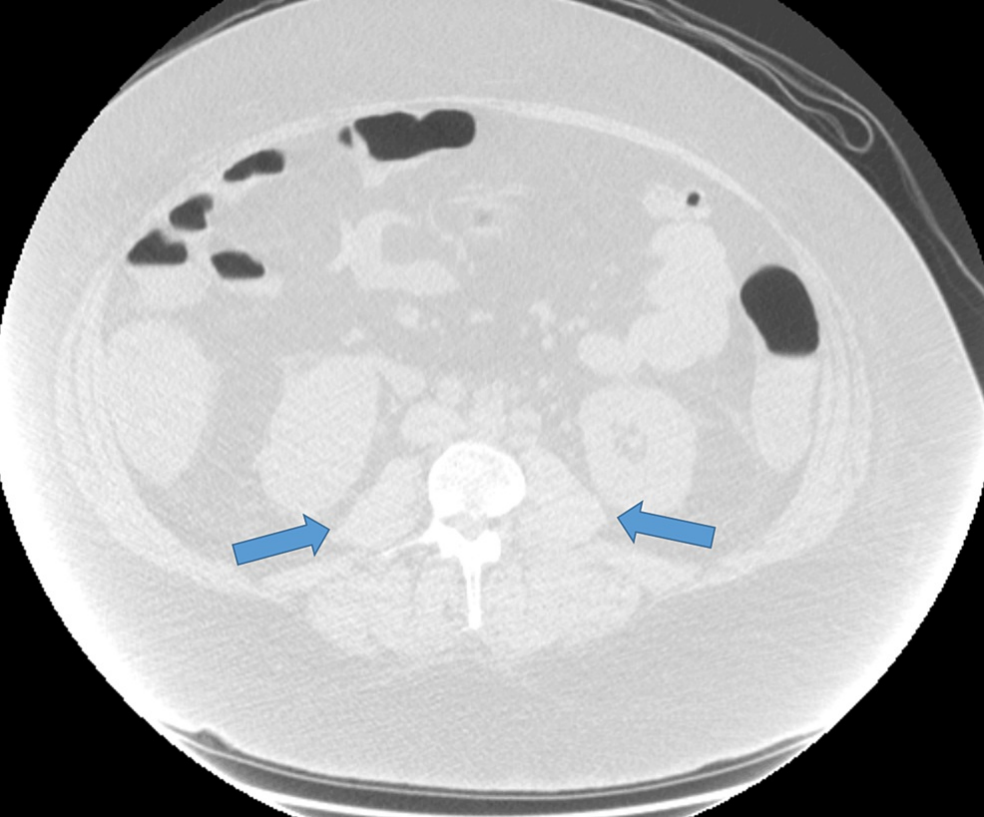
CT abdomen and pelvis showing periportal and mild retroperitoneal lymphadenopathy (blue arrows) CT: computed tomography
